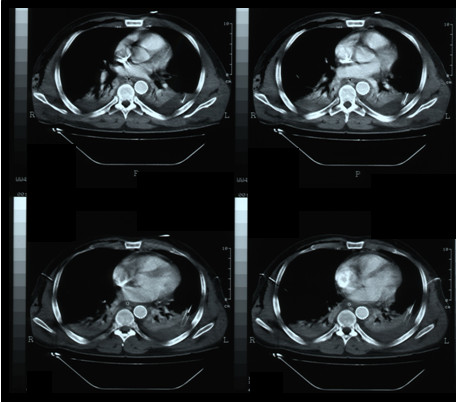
CT scan at 2^nd ^postoperative day. The images show good apposition of the graft to the aortic wall, with no signs of endoleak.
